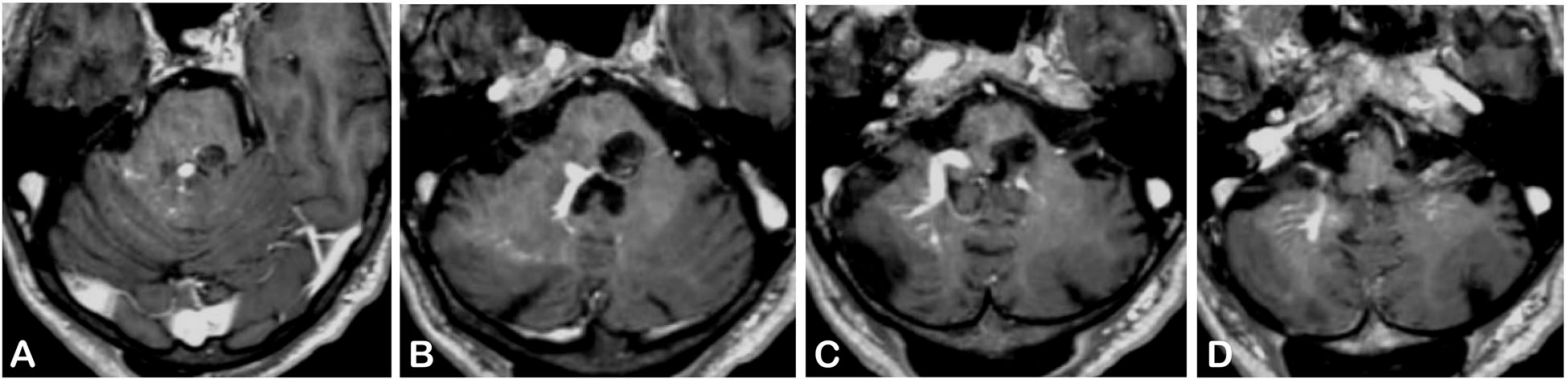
Postoperative MRI of the brain in post-contrast T1-weighted sequence. We performed (d) a suboccipital telovelar approach to gain access to the rhomboid fossa. Then we used (c) the infrafacial triangle as an entry point to the pons to perform microsurgical excision of (a) the BCM and (b,c) the pontine hematoma. a,b,c,d The DVA was left intact
